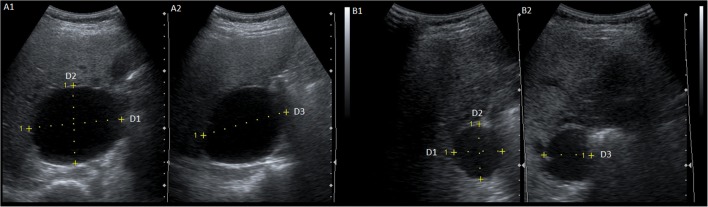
Example of ultrasound measurement of cyst volume at baseline (A, 220 mL) and after 6 months (B, 20 mL). Cyst volume was calculated by multiplying the mean orthogonal diameters using the ellipsoid formula (D1 * D2 * D3 * 0.523). In D1, we measured the cysts medio-lateral direction, D2 dorso-ventral direction, and D3 in cranio-caudal direction
